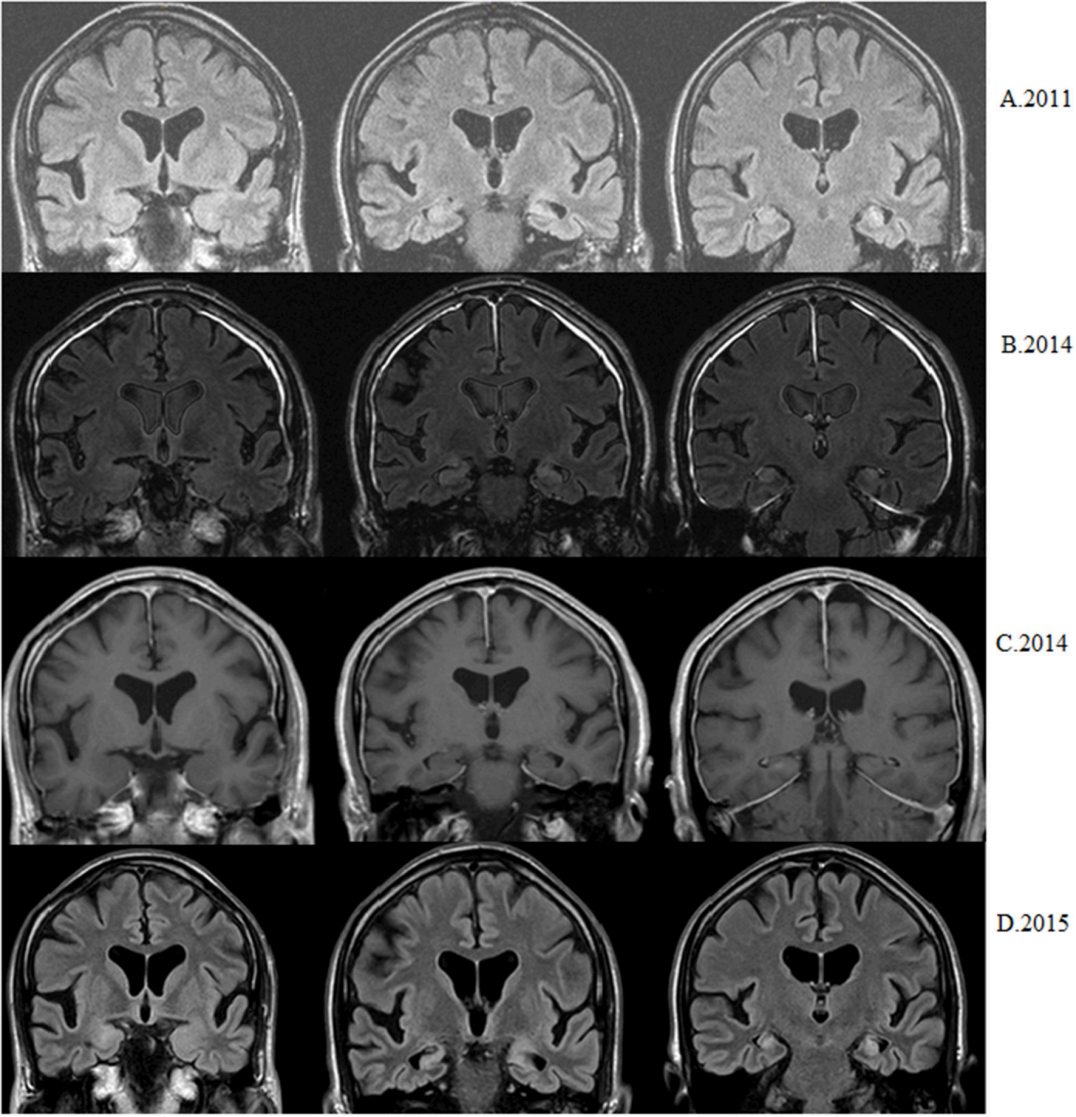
Patient with epilepsy debut at 56. Nowadays seizure-free but with progressive memory decline. (A) First available MRI shows left hippocampal atrophy and hyperintensity (coronal flair). (B) Three years later bilateral hippocampal hyperintensities are observed without right hippocampal atrophy (coronal flair). (C) Three years later nodular right hippocampal contrast enhancement is observed (coronal T1 after injection of gadolinium). (D) After 6 months bilateral hippocampal sclerosis (atrophy+ hyperintensity) is observed (coronal flair).
